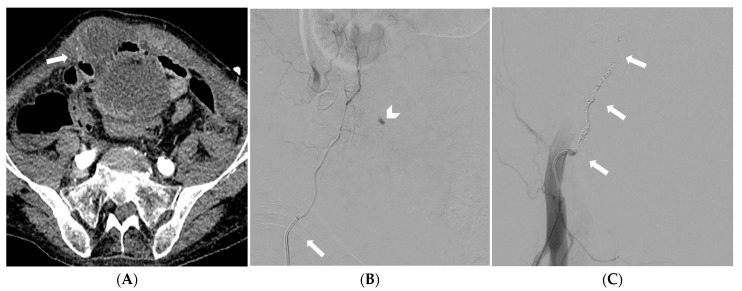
CT angiography depicting a spontaneous rectus sheath hematoma (arrow) (A). Selective catheterization of the right inferior epigastric artery (arrow) with a pseudoaneurysm confirmed at digital subtraction angiography (arrowhead) (B). Digital subtraction angiography showing effective embolization with coil (arrows) embolization starting distal to a small pseudoaneurysm-feeding vessel, thus preventing “backdoor” bleeding (C).
